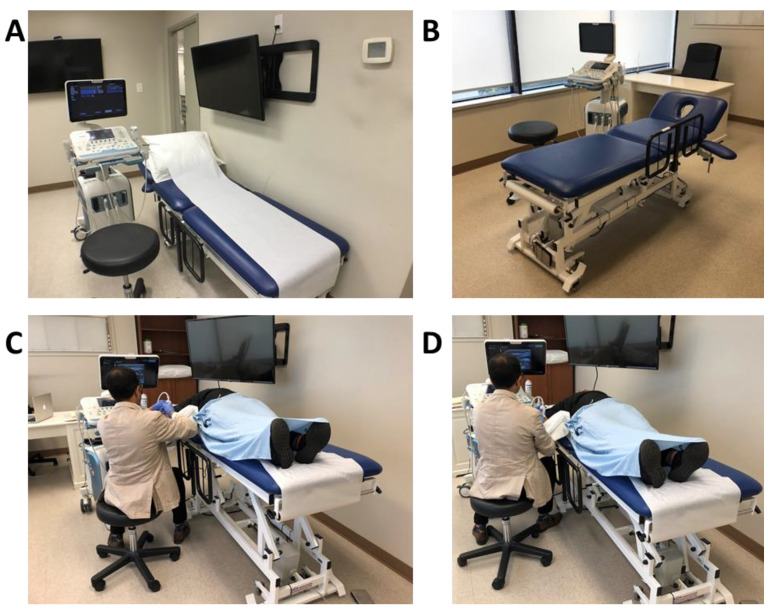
Workstation ergonomics. (A) Typical setup of an injection room with the examination table against the wall. In this situation, if a patient requires bilateral injections, it would potentially require the patient to be turned to access the limb closer to the wall. In addition, note the second screen on the wall to help with visualization. (B) Ideal workstation setup with examination table centered in the middle of the room with adequate space to move ultrasound machine. (C) Suboptimal positioning with a low chair and elevated table result in hiking of the right shoulder and increased strain on the physician’s shoulder. (D) Bed height and swivel stool height are at an optimal level to allow for the upper extremity to fall naturally and the shoulder to be relaxed. The position of ultrasound is at eye level, also resulting in a comfortable and neutral position of the cervical spine.
